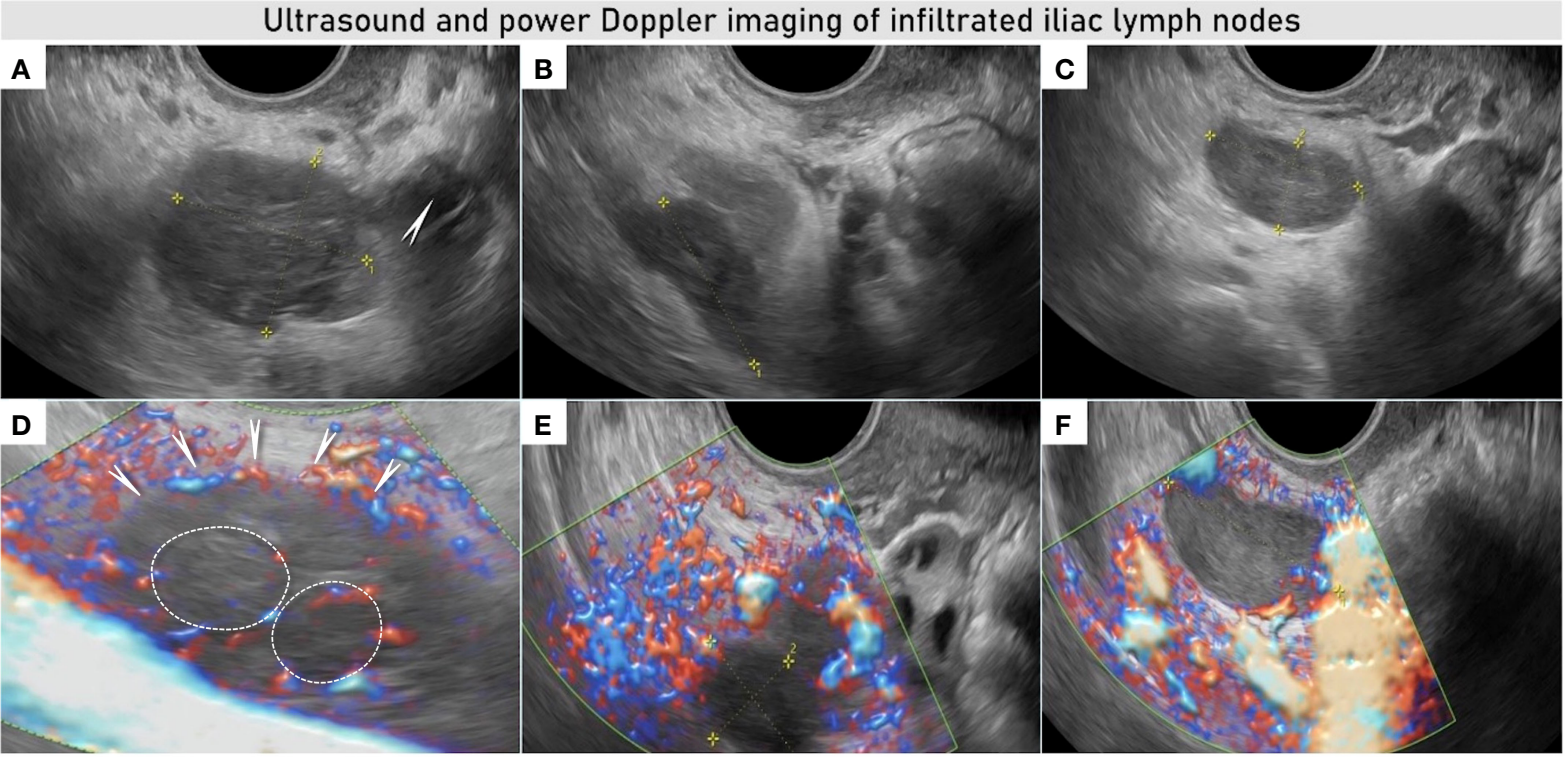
Ultrasound and power Doppler imaging of infiltrated lymph nodes in the right obturator fossa. Transvaginal ultrasound imaging demonstrates three bulky lymph nodes: first iliac lymph node (29 × 26 × 31 mm) infiltrated by tumor nodules located in the close proximity to the right ovary (arrow) (A) with visible transcapsular vessels penetrating the lymph node from the outside (arrows) and ring-shaped vessels marked with white circles (D). Second iliac lymph node (25 × 13 × 21 mm) was lateral to the first one, infiltrating the pelvic side wall and internal iliac vessels up to the interiliac bifurcation with infiltration of the external iliac vein (B), this lymph node was less vascularized on color Doppler images (E). Third infiltrated node (26 × 13 × 30 mm) was located deeper in the pelvis, near the right lateral parametrium (C), with transcapsular vessels on color Doppler image (F) See also Videoclip 1.
